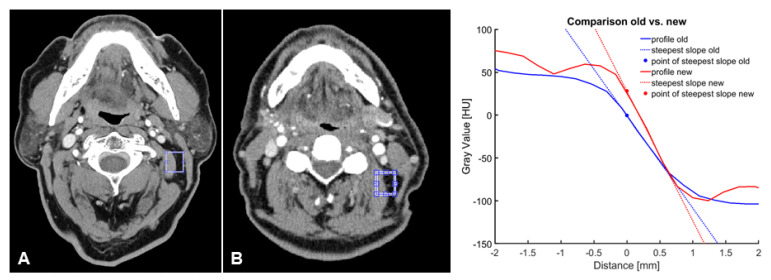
Comparison of contrast-to-noise-ratio of (A) UHR-CT and (B) NR-CT in a 57-year-old male patient with a squamous cell carcinoma of the left upper jaw.
